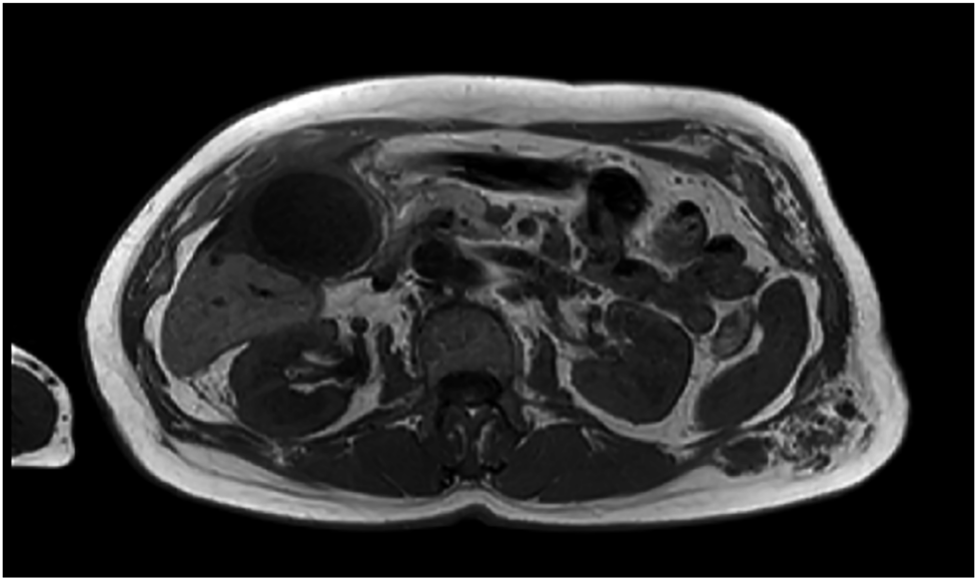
Angio-MRI (transversal scan of the upper abdomen – gall bladder: In addition to the enlarged gall bladder [hydrops]) including a thickened wall → hemangioendothelioma at the left ventral and dorsal wall of the trunk.
